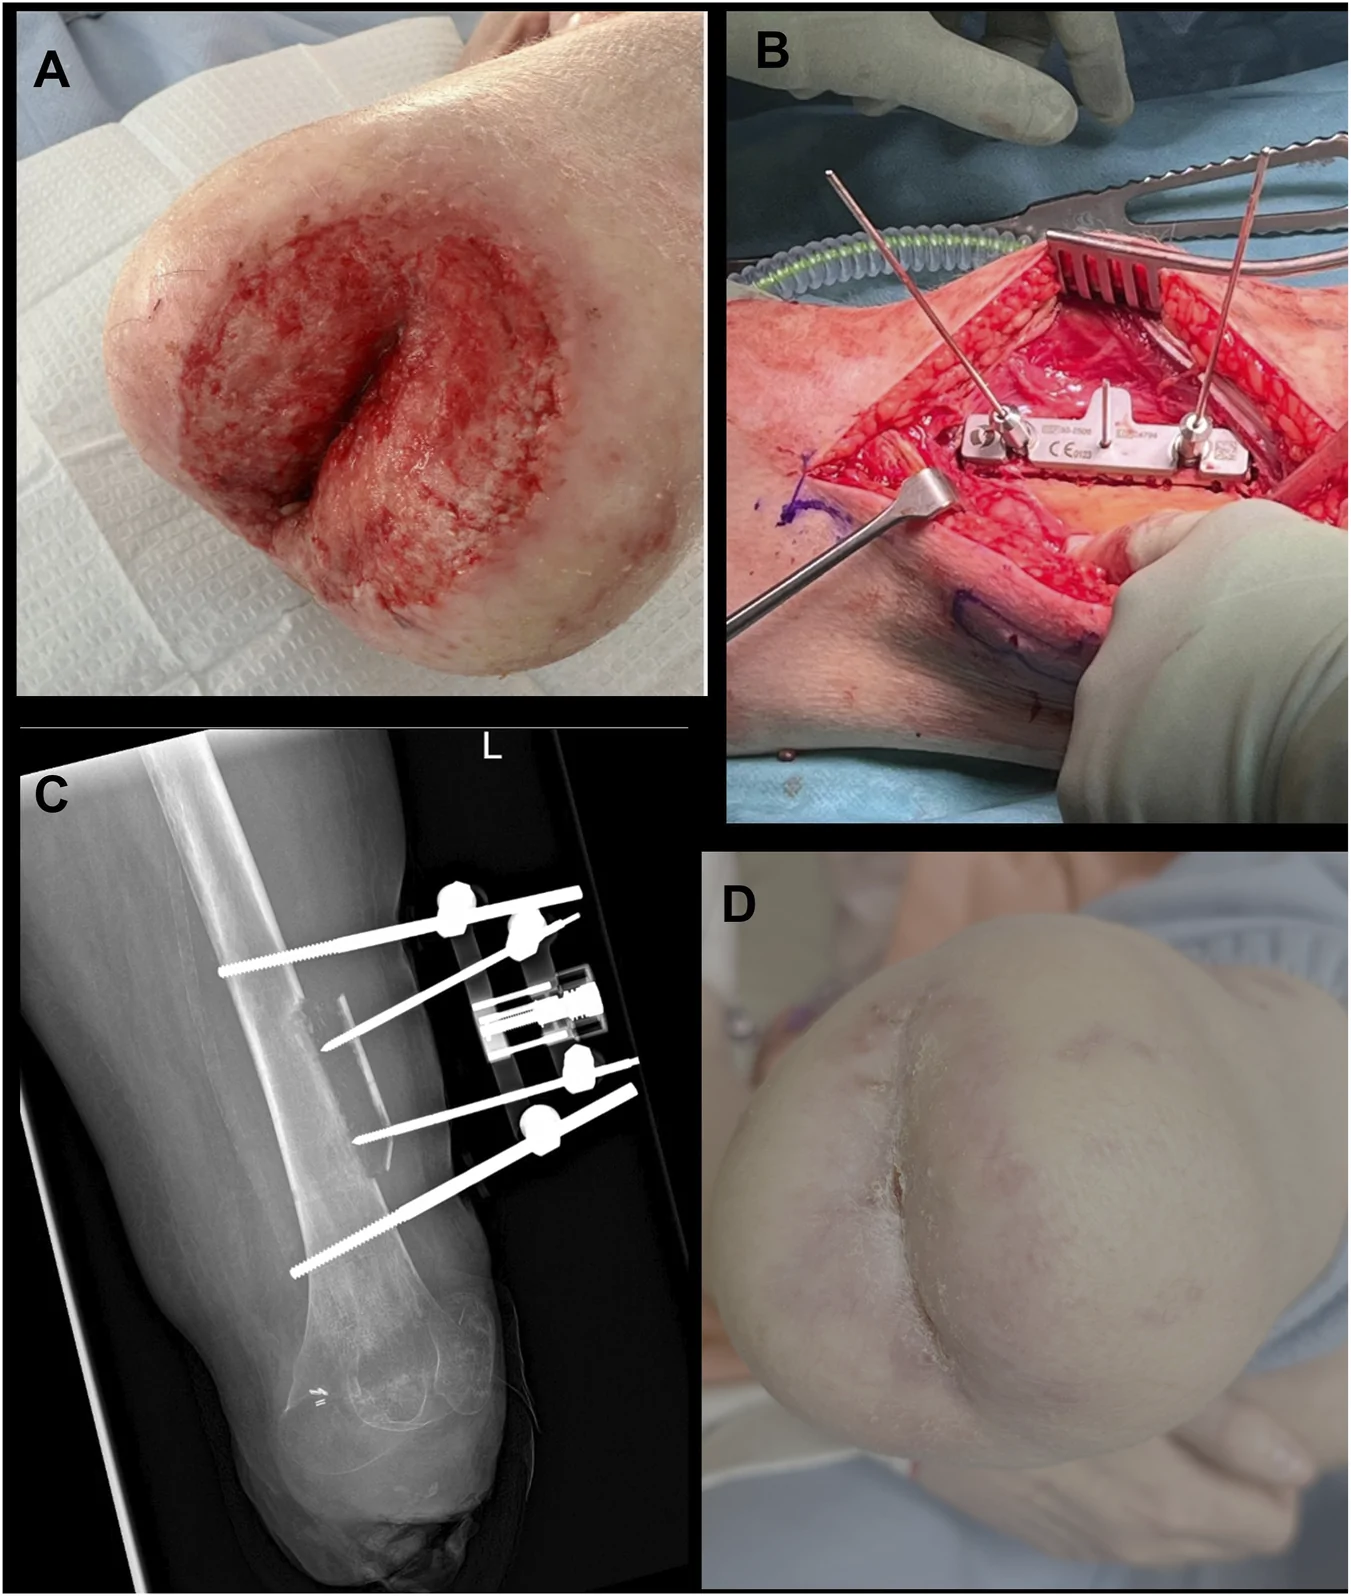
Application of transverse femoral bone transport for stump salvage. Fig. 1-A Preoperative view of the nonhealing, atrophic distal stump defect. Fig. 1-B Intraoperative view of the lateral femoral corticotomy and external fixator application. Fig. 1-C Radiograph during the distraction phase demonstrating elevation of the lateral cortex. Fig. 1-D Final clinical outcome showing a fully healed, stable stump (8 months postoperatively).
